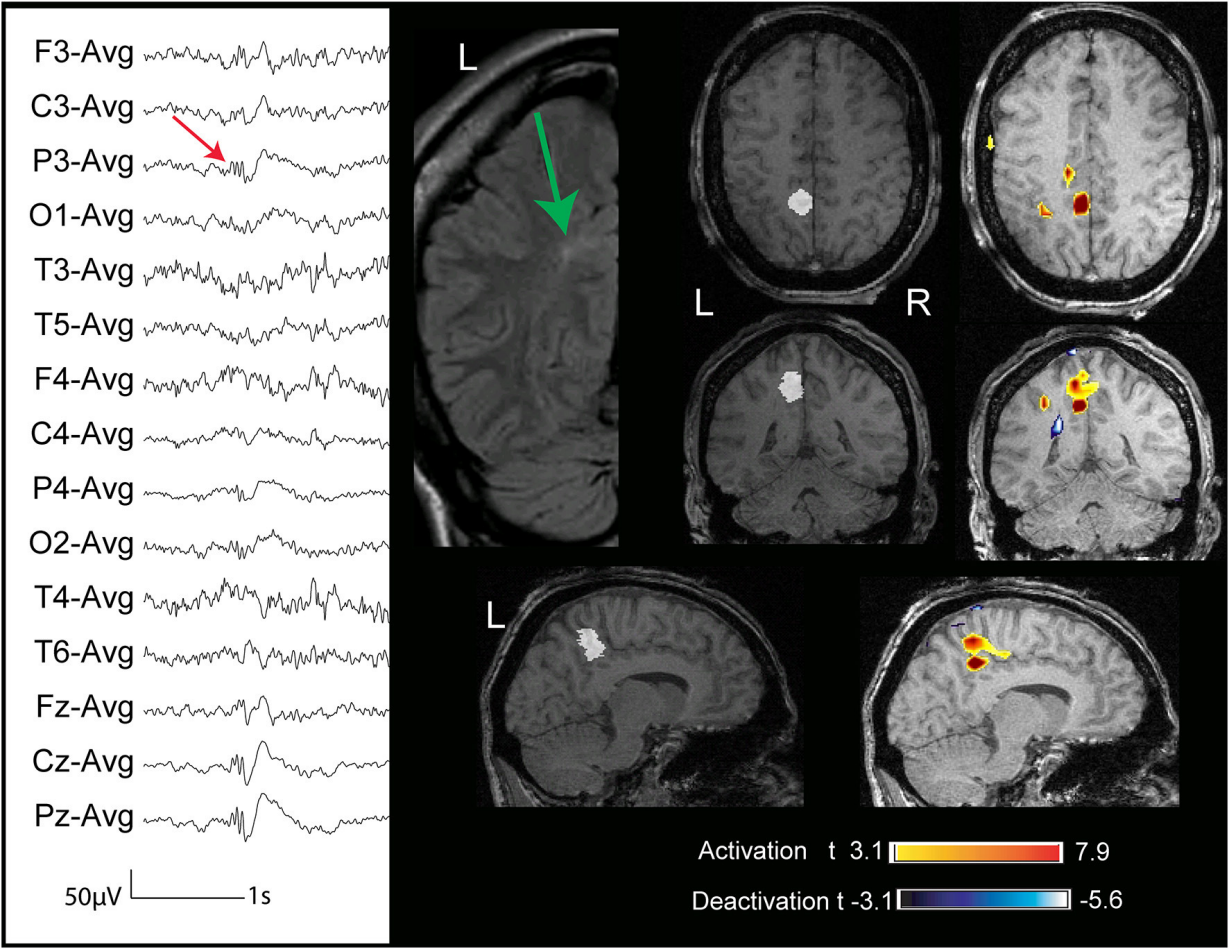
Thirty four year old patient (case 2) with FCD (marked in white) in the paramedial portion of the left precuneus (the green arrow indicates the lesion in the coronal FLAIR sequence). The marked events were polyspikes max at CzPzP3 (average reference). The BOLD response showed a focal activation with a maximum *t*-value in the lesion.
